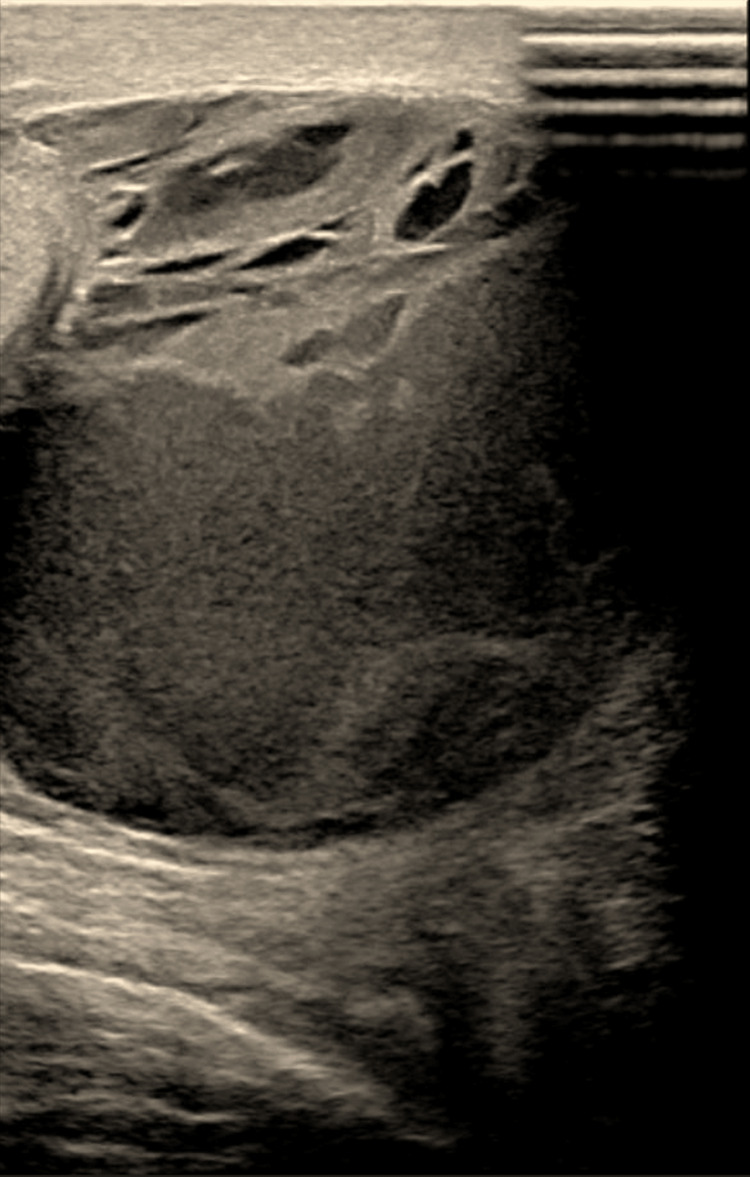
The scrotal ultrasound shows a loculated turbid fluid collection surrounding the right testicle with an internal thick hyperechoic septation; the findings are suggestive of a right-sided scrotal abscess.
